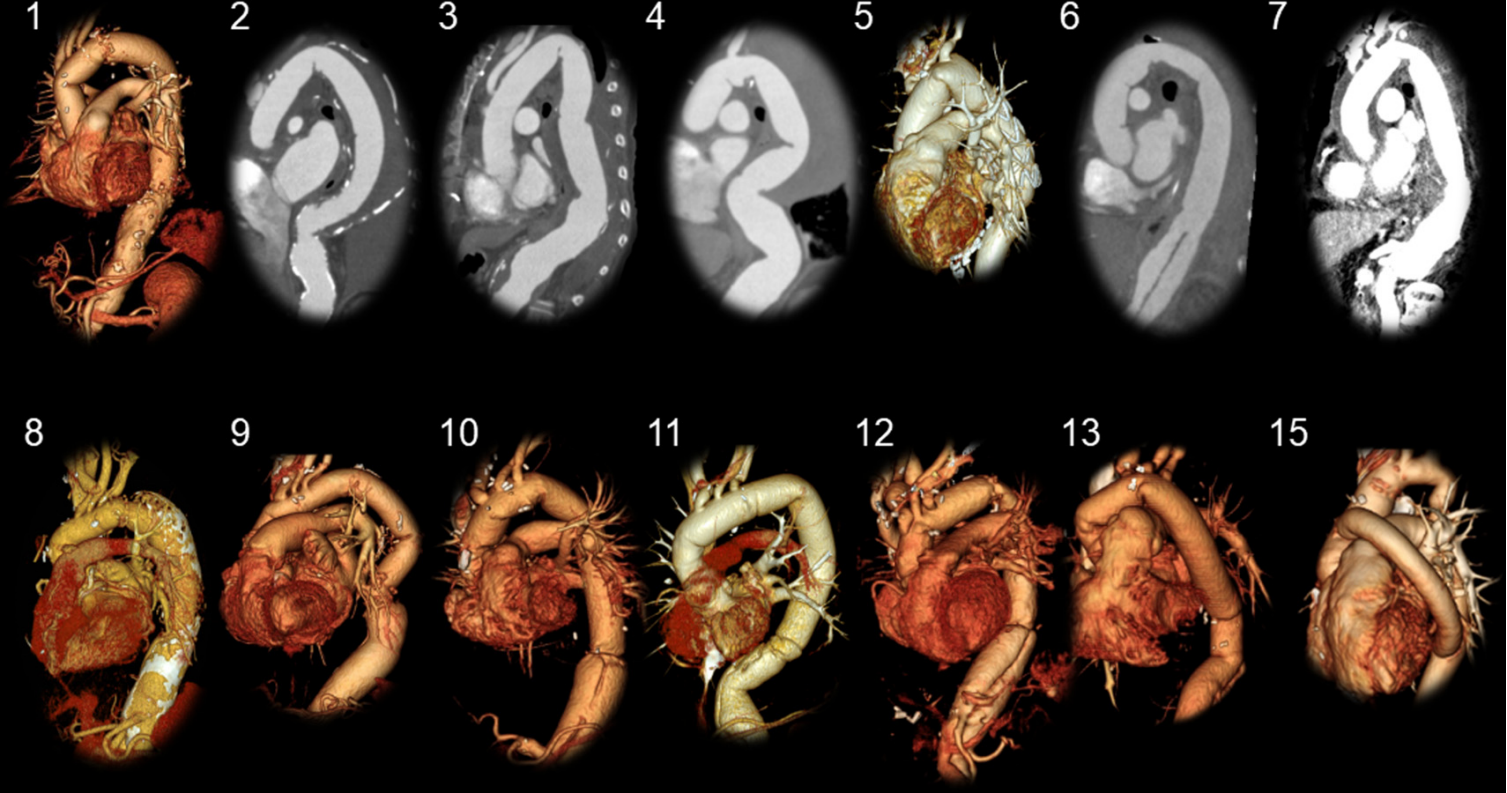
Postoperative computed tomography scan images taken within 14 days after the operation.
